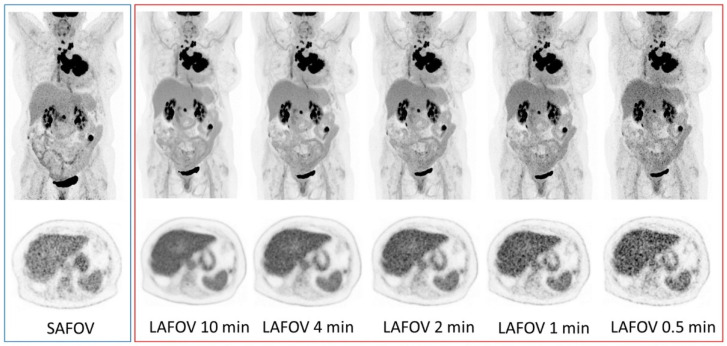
Representative maximum intensity projection (MIP, top row) and axial PET images (bottom row) of a 57-year-old female with non-small-cell lung cancer. Presented are images for the standard axial field-of-view acquisitions on the left (blue margin) and the large axial field-of-view for 10-, 4-, 2-, 1- and 0.5-min acquisitions (right, red margin). For reference, the PET window was set to 0 to 8.5 SUV. Image from Alberts et al.; Eur. J. Nucl. Med. Mol. Imaging [27].
